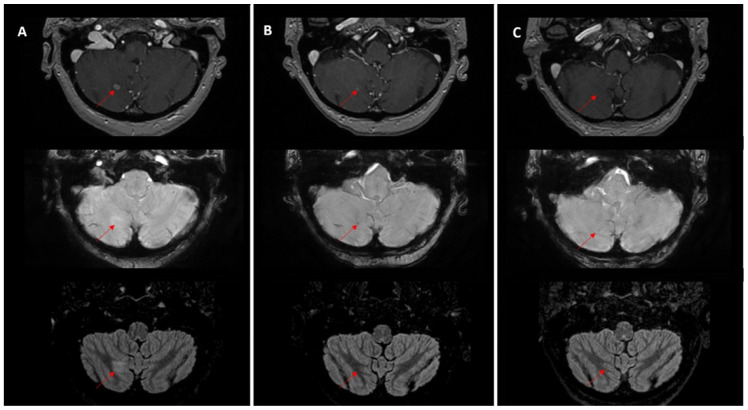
Evolution of a right cerebellar metastasis (red arrows) in a patient with breast cancer and multiple secondary lesions. (A) Before treatment MRI showed a 6 mm right cerebellar lesion with ring enhancement on post-contrast T1, no susceptibility artifacts on SWAN and hyperintense on T2/FLAIR. (B) At 8 weeks after whole-brain radiotherapy (30 Gy/10 fractions) with a sequential boost up to 45 Gy to the cerebellar lesion, there was dimensional regression with residual millimetric enhancement, the appearance of a hypointense susceptibility artifact on SWAN, and resolution of signal abnormalities on FLAIR. (C) At 6 months after radiotherapy, the lesion was no longer visible on conventional sequences; only a subtle punctiform hypointense focus on SWAN could be appreciated, which would not be recognized as a treated metastasis in the absence of longitudinal tracking.
